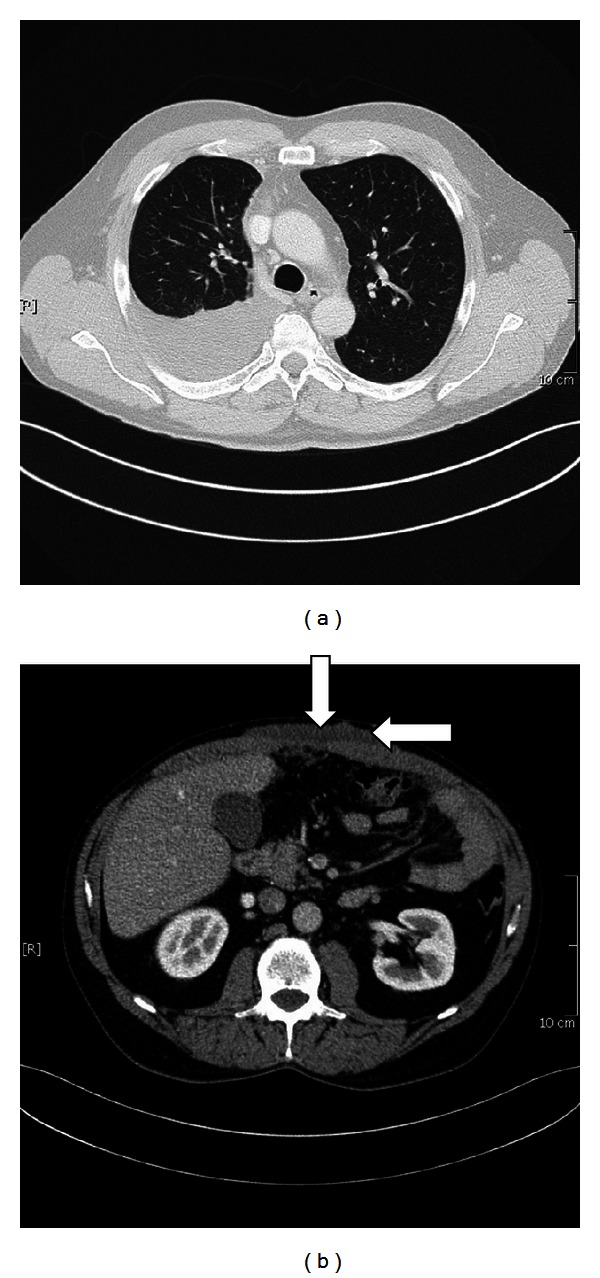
(a) CT scan shows a pleural effusion; the surface of the right lung is colonized and infiltrated by pleural mesothelioma. (b) Large tumor mass of the abdominal wall (10 × 7 cm) without penetration into the peritoneum.
